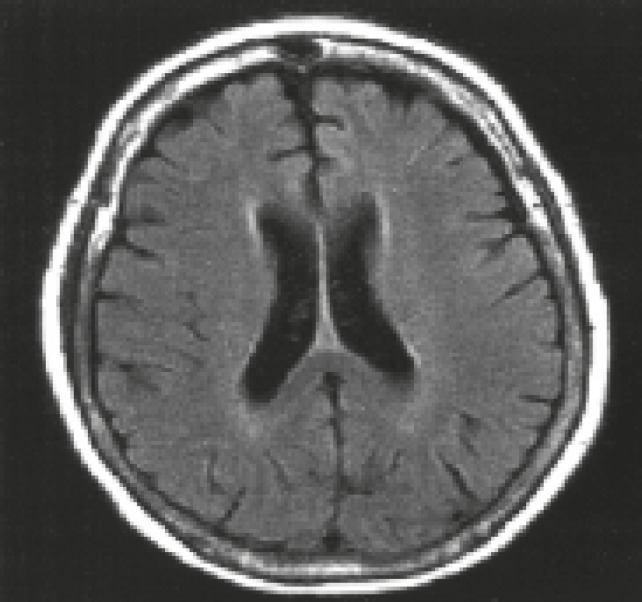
FLAIR MRI demonstrating resolution of hypersignal zones
